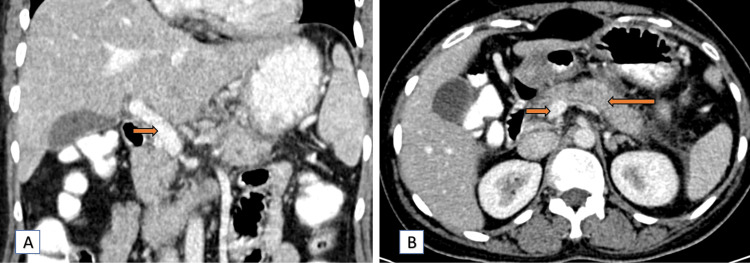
Contrast CT images (coronal and axial) revealing normal contrast opacification in the portal vein and splenic vein Contrast CT images (portal phase) reveal good contrast opacification of the portal vein at the region of the portal vein confluence in coronal (short arrow) (A) and axial (short arrow) (B) sections, with normal pancreatic parenchymal attenuation in the axial (long arrow) (B) section. Contrast CT images (arterial phase) reveal good contrast opacification of the splenic vein in coronal (blue arrow) (C) and axial (blue arrow) (D) sections. CT: computed tomography
